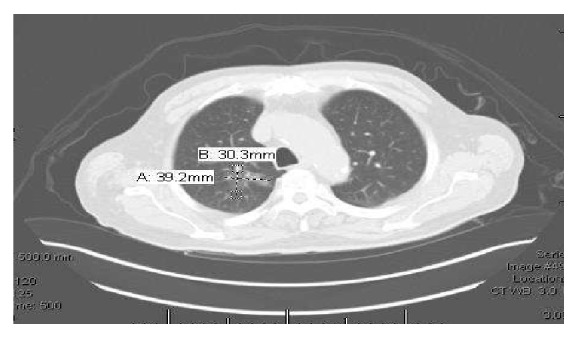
CT scan showing a 3.9 × 3 cm mass in upper lobe of right lung.
